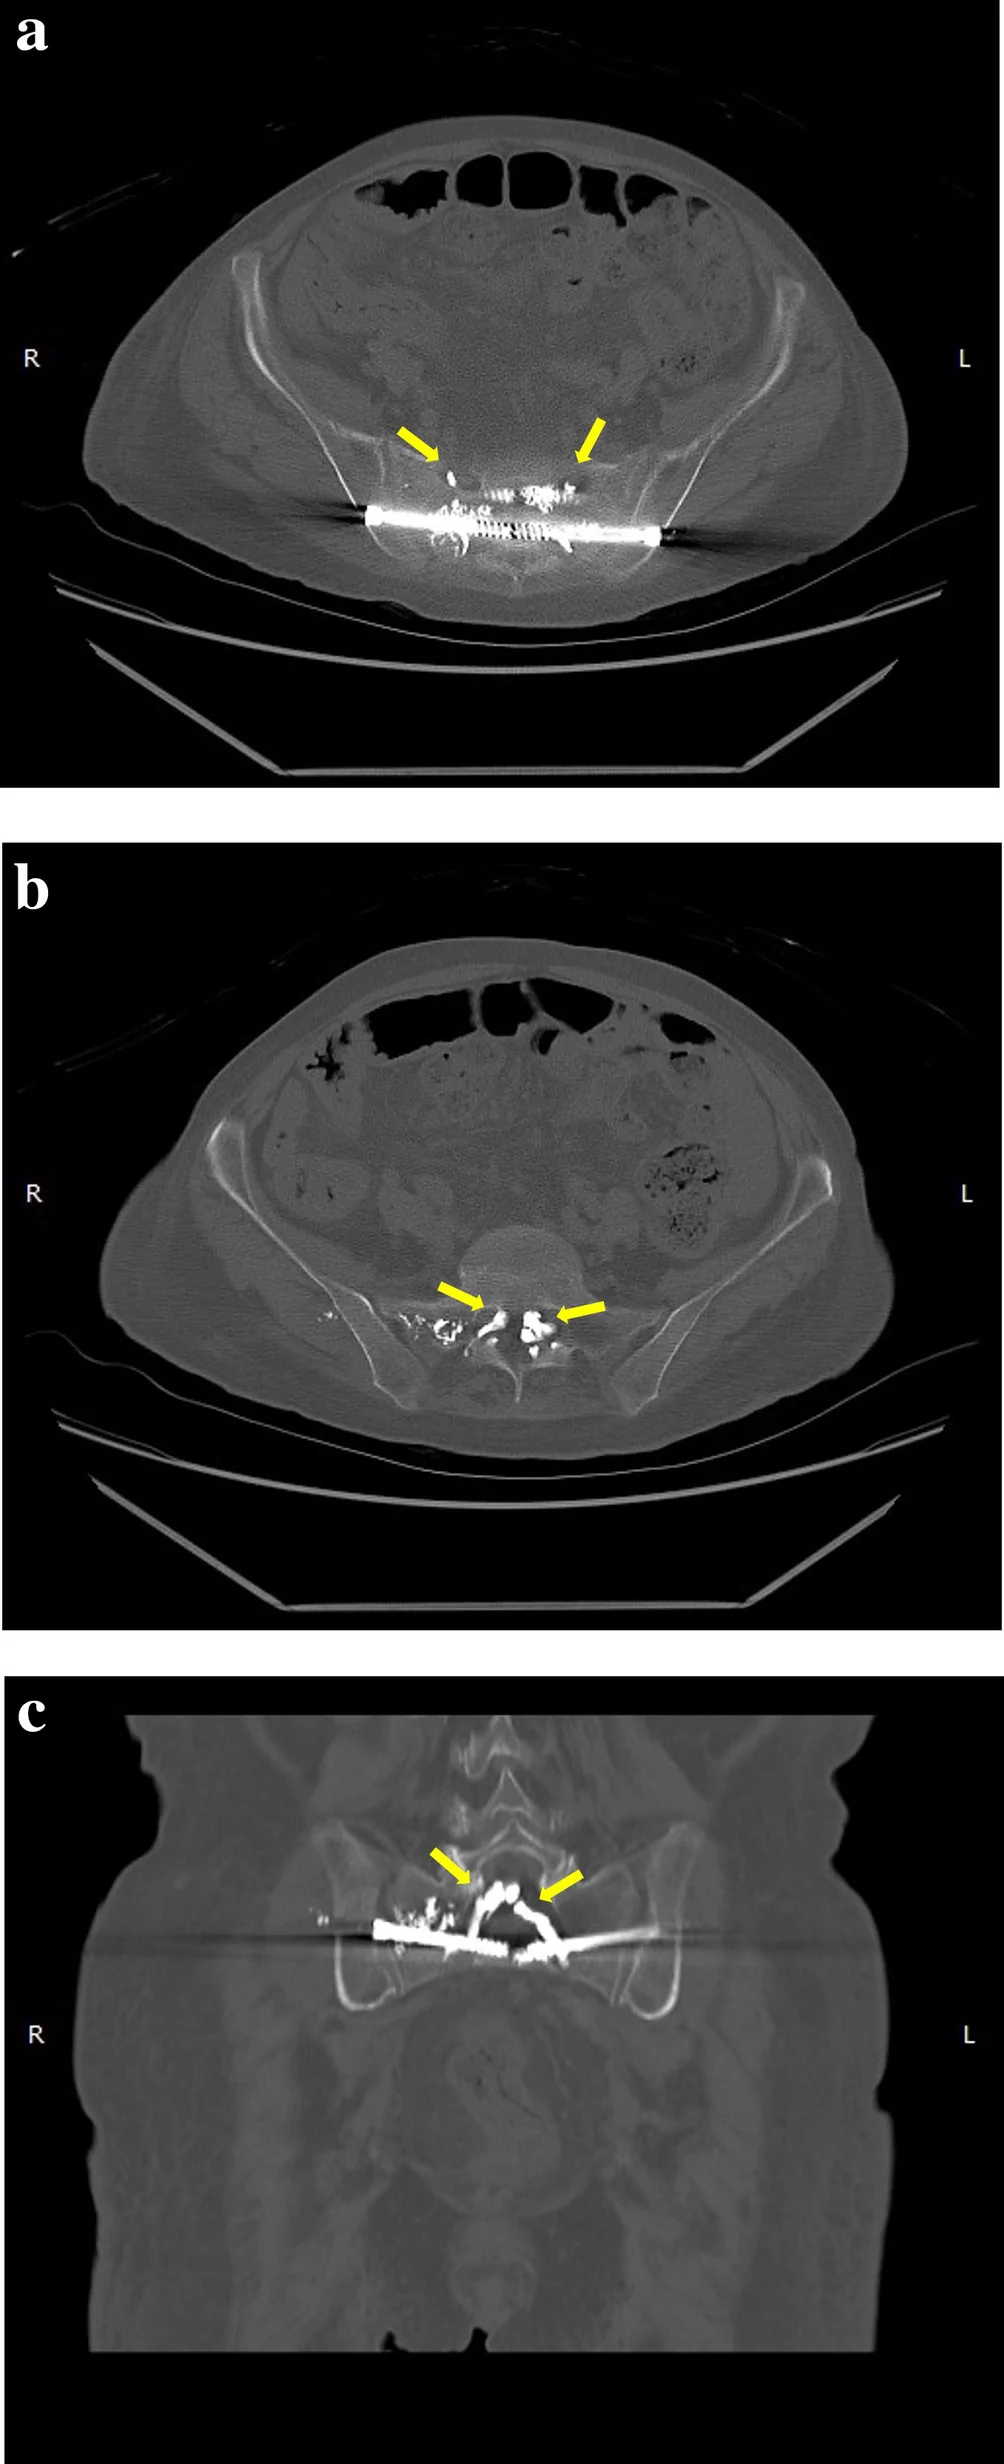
CT image showing unexpected cement extravasation into spinal canal (yellow arrows) after SI screw fixation and sacroplasty in axial view (a, b) and coronal view (c).
